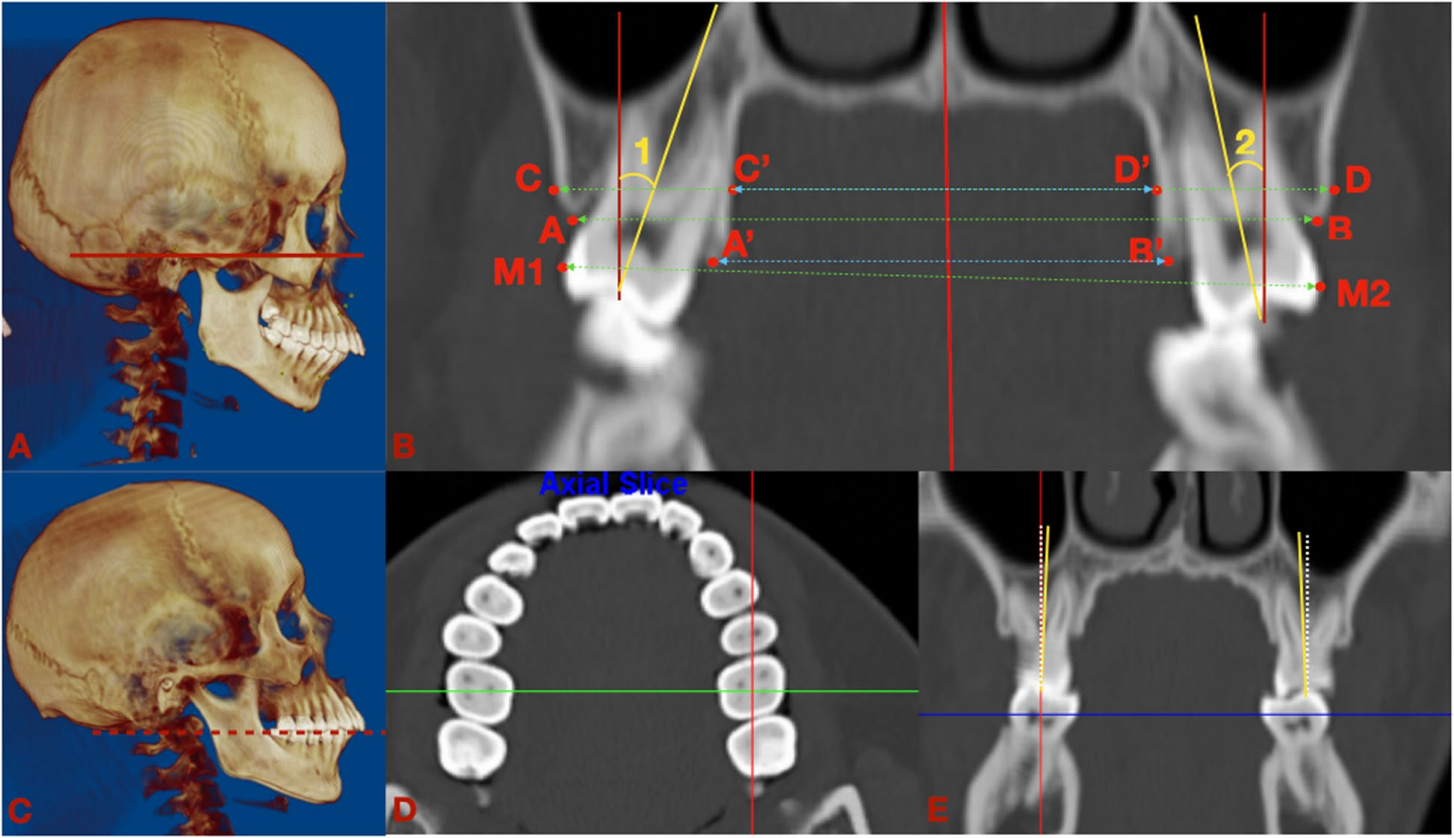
The demography of transverse measurements on the upper first molar. A: measured when the FH plane was parallel to the floor; B: Linear and angular measurements of upper first molar on the coronal CT image. A-B: U6-AC; C-D: U6-MR; M1-M2: U6-IM; (AB-A’B’)/2: U6-BLW; (CD-C’D’)/2: U6MR-BLW; 1: ∠16/Sagittal; 2: L: ∠46/Sagittal; C: measured when the OP was parallel to the floor; D:on the axial section of maxillary arch, the coronal slide was defined as a line (green line) connecting the midpoint of the mesiodistal occlusal crown width of bilateral first molars; E: the long axis of upper first molar was defined as a line (yellow line) passing through the midpoint of the buccal and lingual cusp tips and the midpoint of the buccolingual width at the cervical base close to the furcation of the anatomic crown. The BLI of the tooth was the angle formed by the long axis of molar and true vertical line
